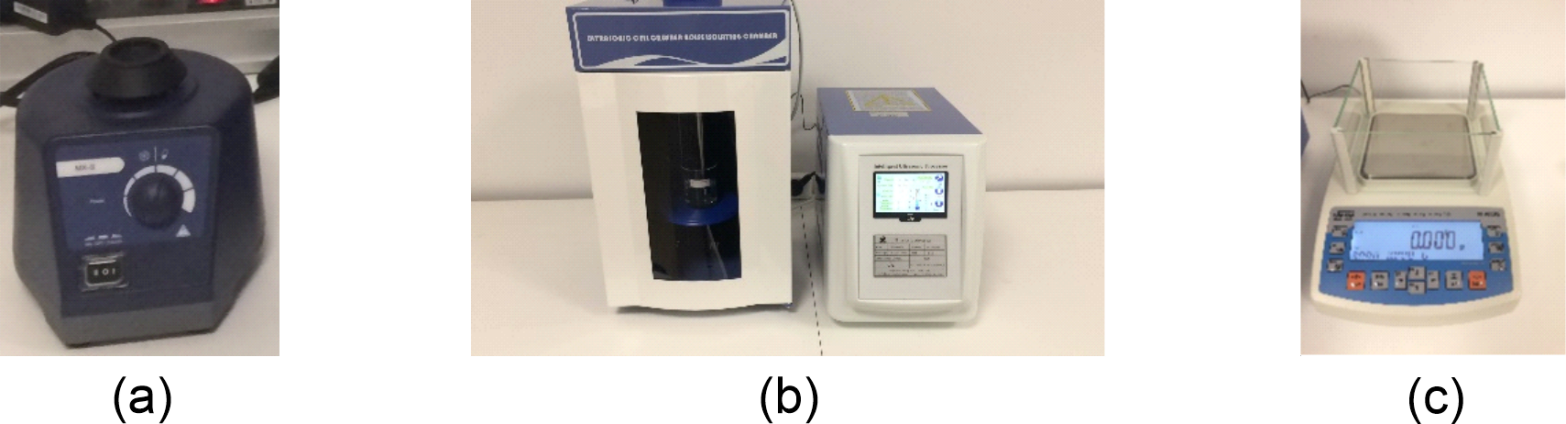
The devices used to implement the two-step method are a) mechanical stirrer, b) ultrasonic stirrer, and c) precision balance.
